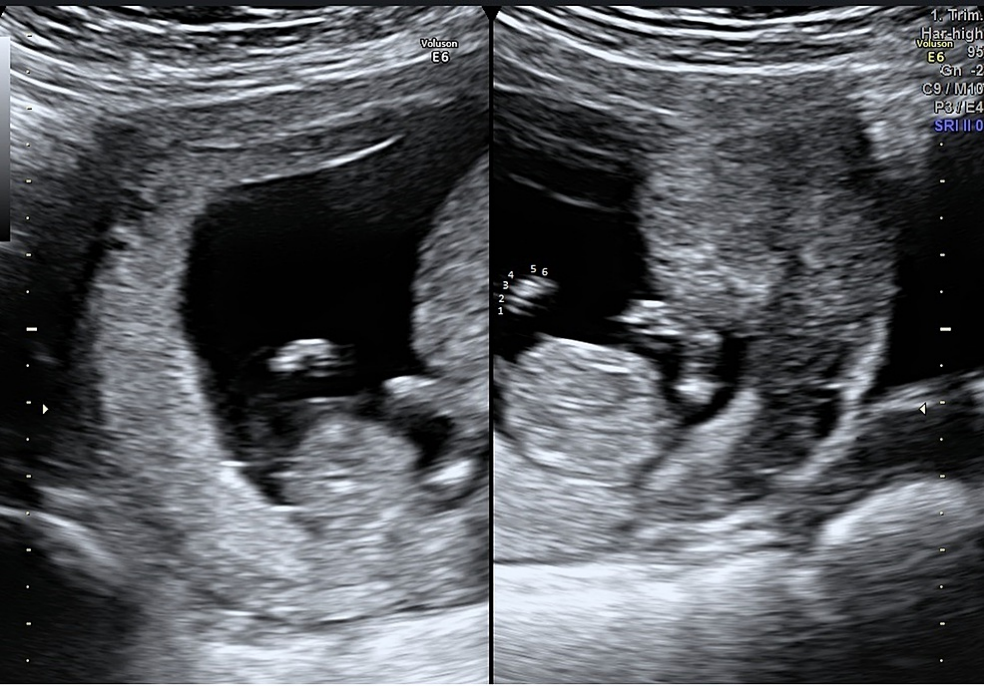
Two-dimensional appearance of hexadactyly on ultrasound.
